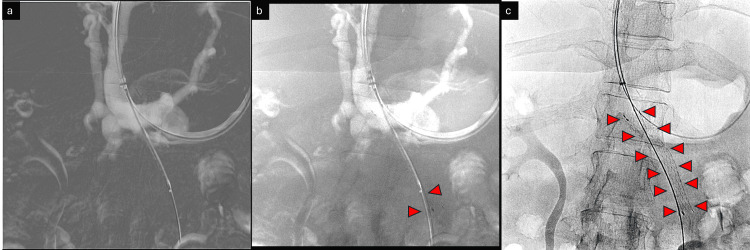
Superior mesenteric vein stenting Images showing stenting of the superior mesenteric vein (SMV). (a) Positioning of an undeployed stent under Road-Map guidance; (b) deployment of stent under Road-Map guidance (distal deployed struts marked with red arrowheads); (c) fully deployed stent (single-shot radiograph image, deployed stent marked with red arrowheads).
